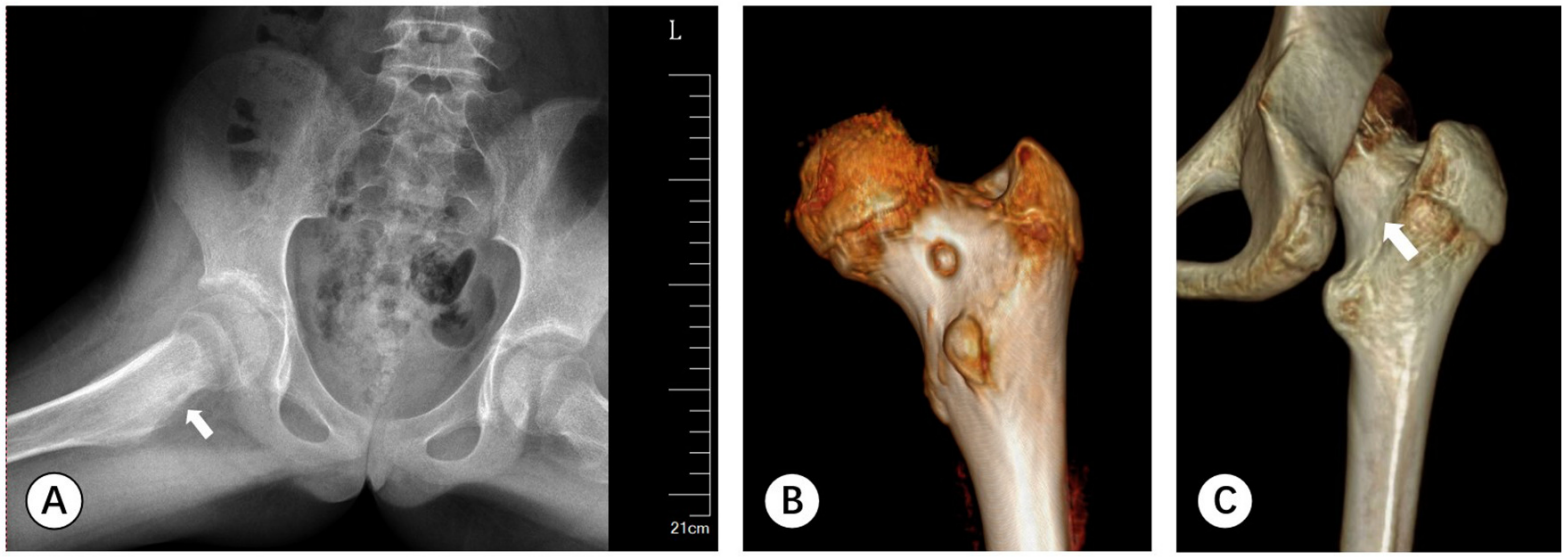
(A) A-P pelvic radiography shows an increase in density of the right femoral neck, with localized circular low-density shadows and slight periosteal reaction around it (white arrow). (B) CT scan of the posterior hip joint reveals a 7 mm circular low-density area visible on the inner side of the femoral neck. (C) Follow-up CT scan depicts the nidus has been completely cleared (white arrow).
